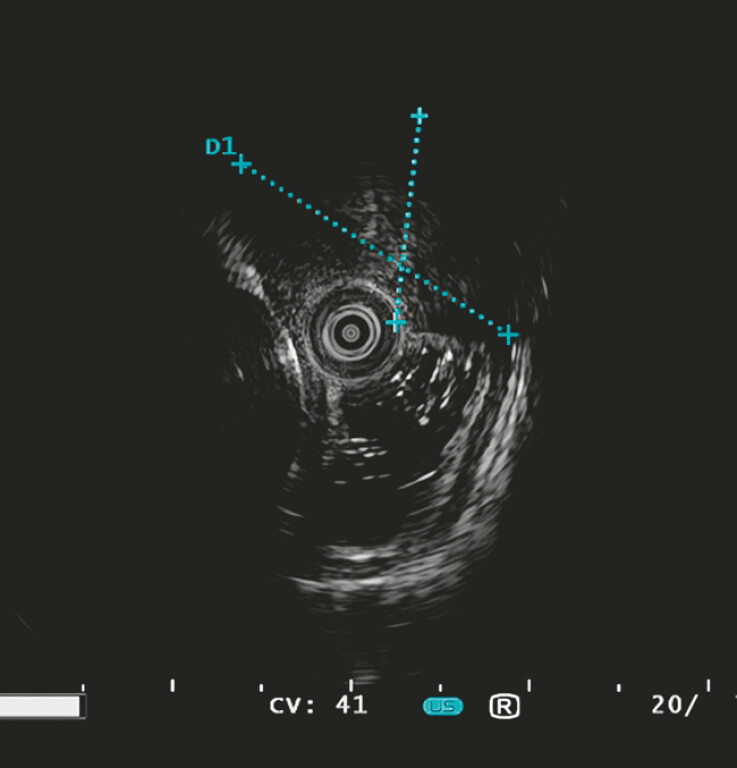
Endoscopic ultrasound image showing a hypoechoic mass protruding into the esophageal and mediastinal cavities, which was poorly demarcated from the muscularis propria.
